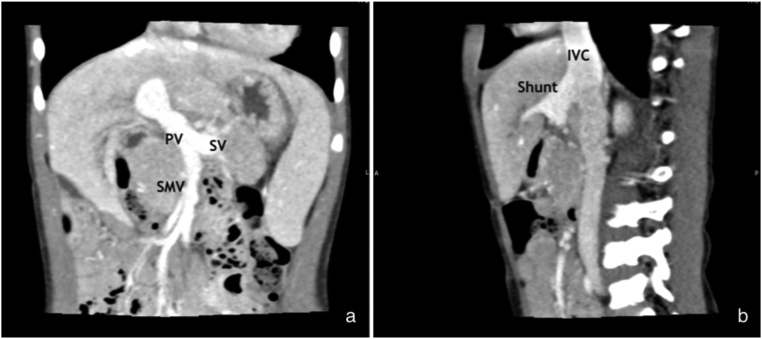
A contrast-enhanced CT scan showed the enlarged main portal vein (PV), splenic vein (SV), superior mesenteric vein (SMV), and a portosystemic shunt connecting the left portal vein to inferior vena cava (IVC).
